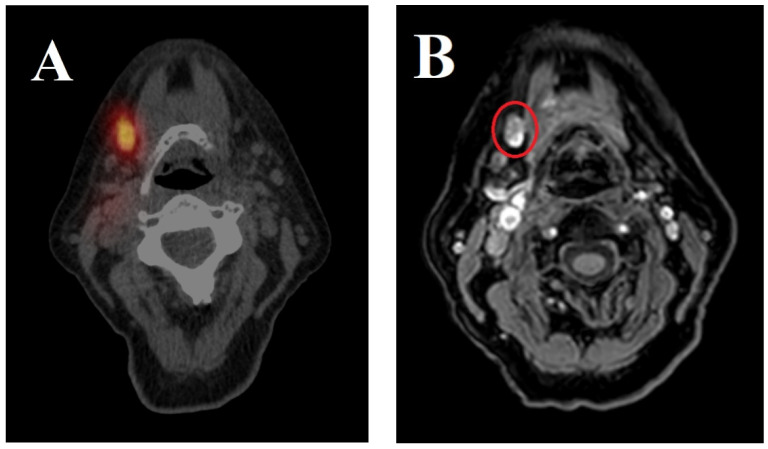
Conventional [^99m^Tc]Tc-nanocolloid lymphoscintigraphy and gadobutrol-enhanced MR lymphography. (A) Conventional [^99m^Tc]Tc-nanocolloid lymphoscintigraphy of a patient diagnosed with cT1N0 OSCC on the right side of the oral tongue (patient 2; Table 2), sentinel lymph node in level Ib on the right side with uptake of [^99m^Tc]Tc-nanocolloid. (B) Gadobutrol-enhanced MR lymphography for the same patient depicting the sentinel lymph node in level Ib on the right side (red circle).
